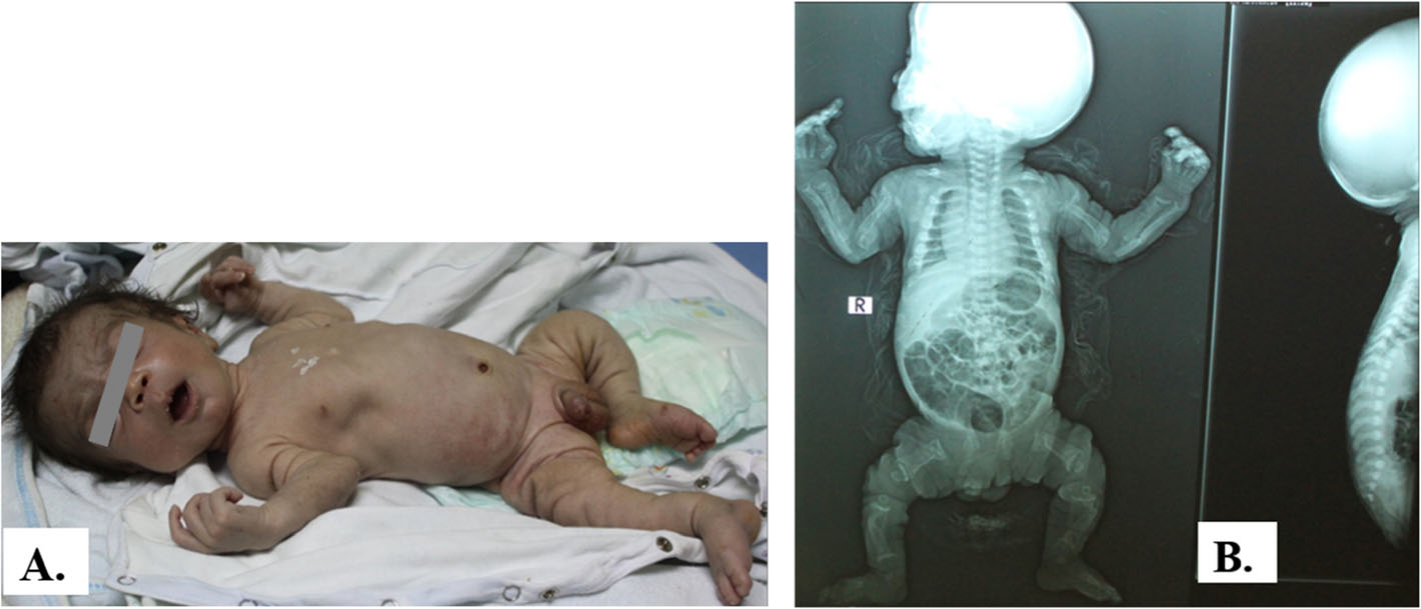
Patient no. 2 at the age of 3 months. A Coarse facies, digital contractures, indrawn chest wall, and Michelin tyre appearance of skin folds in the limbs. B X-rays showing short thick long bones with splayed metaphyses, small epiphyses, horizontal ribs and short, square or biconvex vertebral bodies
